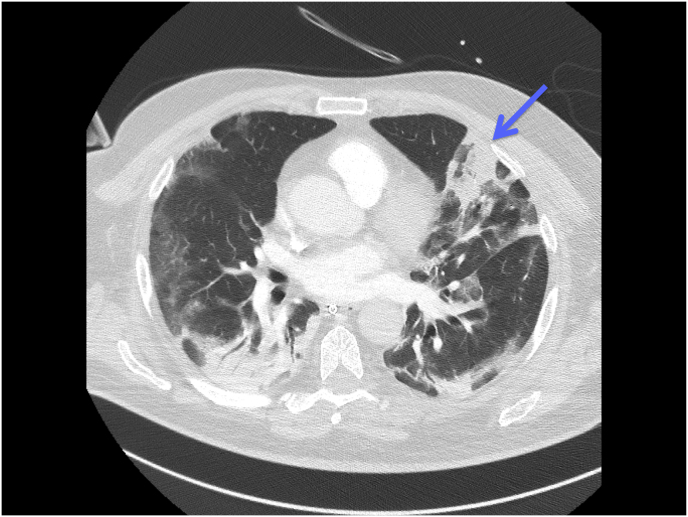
CT scan of the thorax taken on day 14 demonstrates the appearance of consolidation in the upper left pulmonary lobe (blue arrow), extended ground-glass opacity and the crazy-paving pattern associated with posterior lung consolidation. (For interpretation of the references to colour in this figure legend, the reader is referred to the Web version of this article.)
